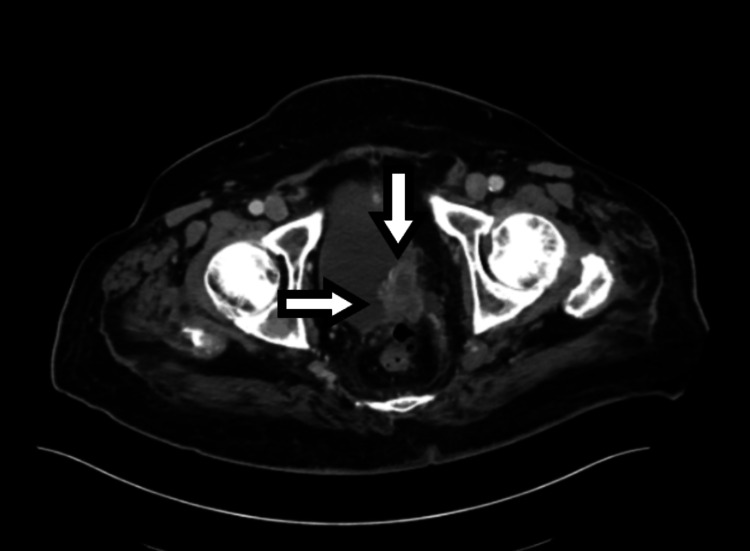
A CT scan of the abdomen and pelvis demonstrated an irregular soft tissue mass at the left posterolateral bladder base, involving the ureterovesical junction, highly suggestive of neoplasm in transverse view CT: computed tomography
